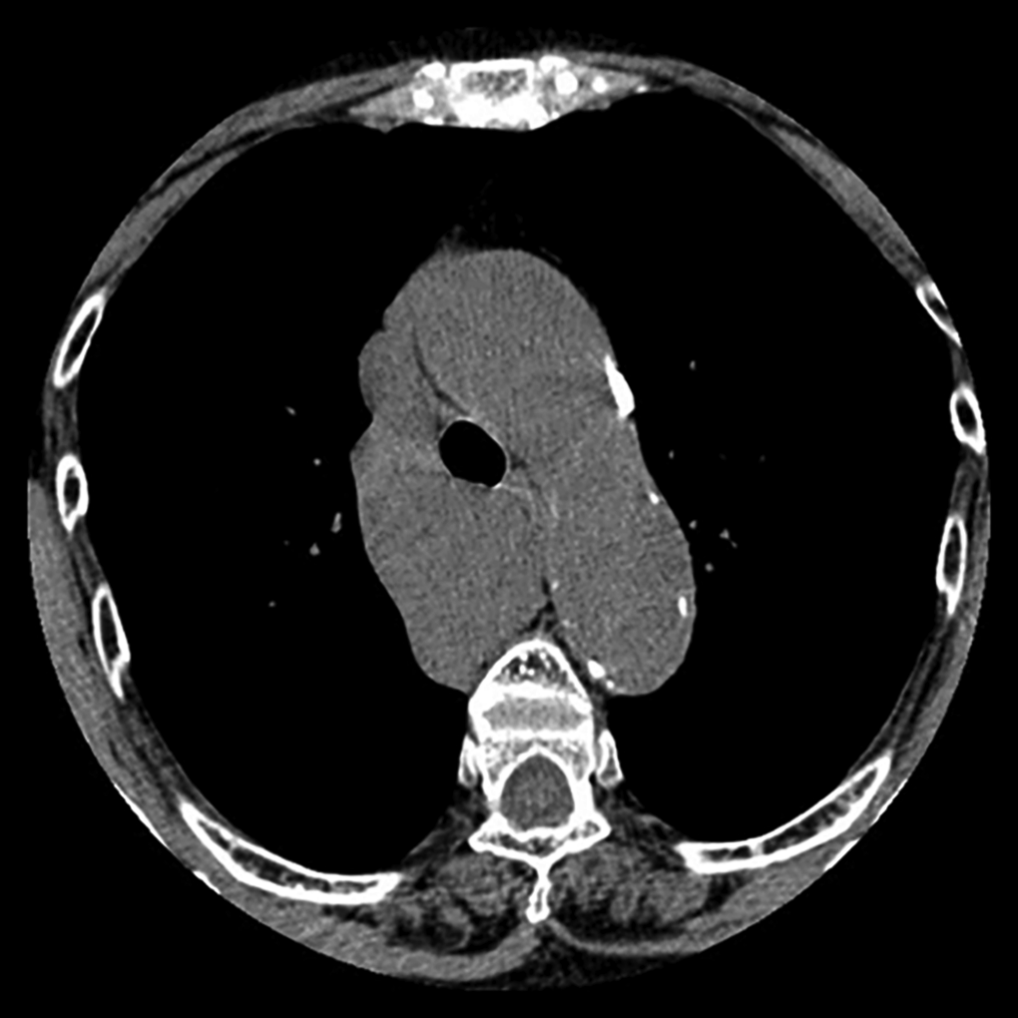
Computed tomography (CT) showed a well‐defined tumour behind the trachea.
